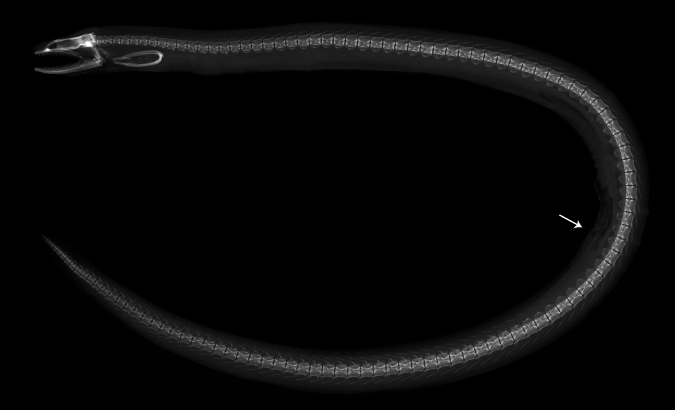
Radiograph showing the skeletal structure of *Uropterygiushades* sp. nov., PNM 15806, paratype, 313 mm TL. Arrow indicates the position of anus.
